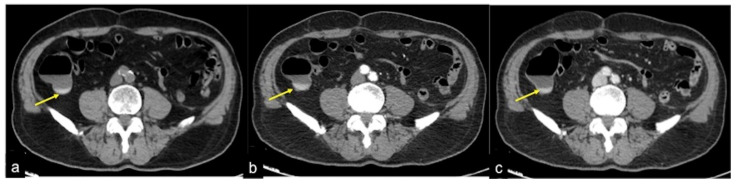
CTA multiphasic study of caecum sentinel clot in a patient with hematochezia. A small amount of spontaneous hyperdensity is collected at caecal fondus, resulting visible in the pre-contrast (a), arterial (b) and venous (c) phases with analogous morphologic and densitometric characteristics (a–c, arrow). This finding is suggestive of a sentinel clot and presumable recent bleeding.
